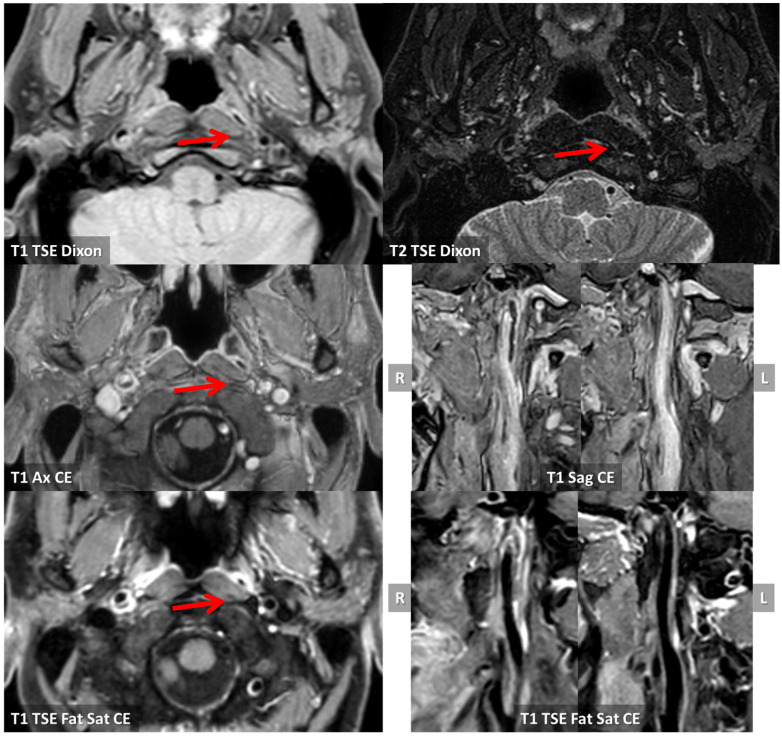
MRI with vessel wall imaging at the level of the right prepetrous ICA. In the first line, T1 and T2 TSE Dixon sequences in axial plane are imaged, showing a hyperintense luminal layer delimiting a hypointense region in the vessel wall in the posterior half (red arrows). In post-contrast T1 sequences, both in axial and sagittal planes (lines 2 and 3 of the figure), no contrast enhancement within the hypointense region is evident, but a vivid peripheral contrast enhancement was found.
